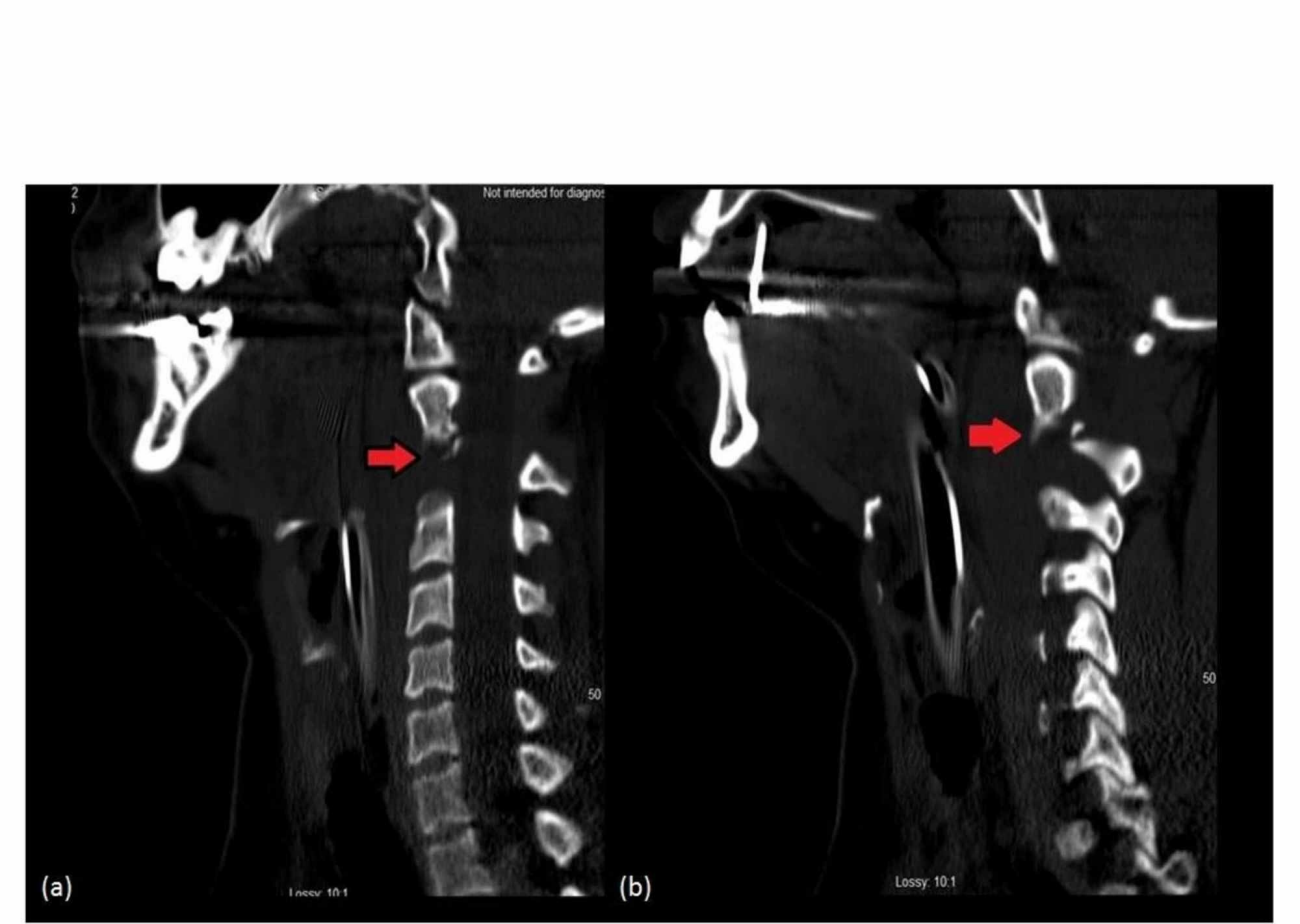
Sagittal view (a) left and (b) right : CT cervical spine with a red arrow showing C2 pedicle fracture.
